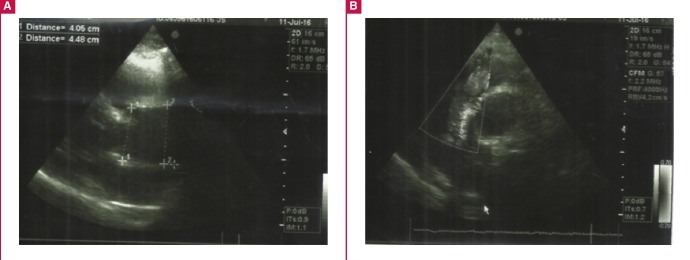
Echocardiography showing dilatation of the ascending aorta.
